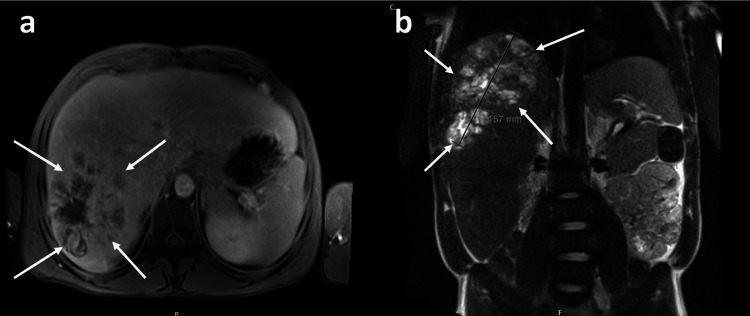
MRI of the liver (a) Axial view: There’s a distinct dark lesion in the liver, which stands out from the surrounding tissue. It is irregular in shape and appears hypointense compared to the normal liver parenchyma and corresponds to a metastatic deposit. (b) Coronal view: The large mass in the liver region is clearly marked with a measurement of 157 mm, suggesting a significant abnormality. Its size and location are consistent with a metastatic GIST.
